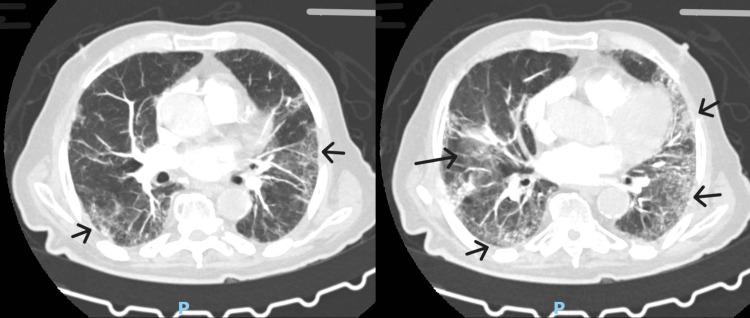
Chest CT done in the ward, showing fibrotic changes predominantly at the lung bases
